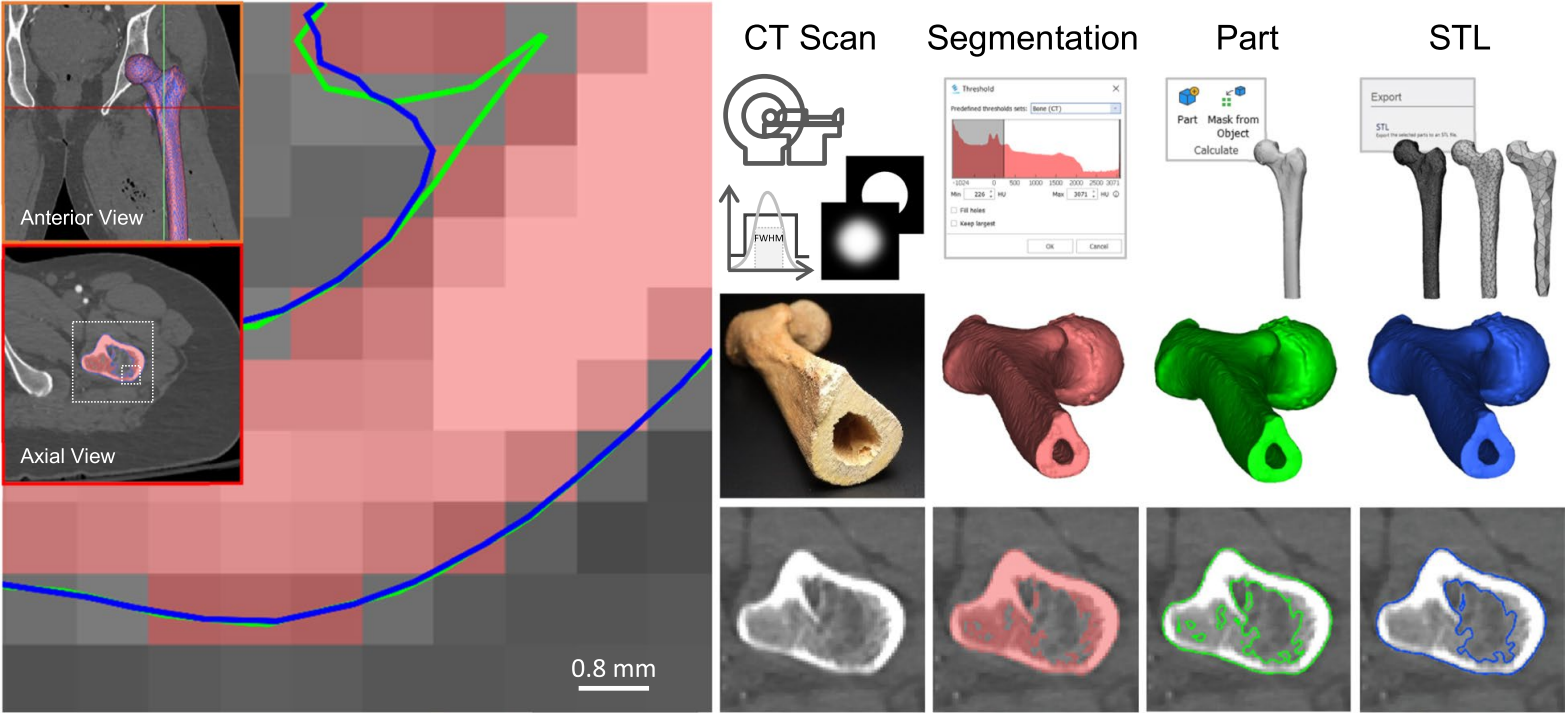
Comparison of 3D model morphology of a femur at high magnification. CT scan data (greyscale) was segmented (red) in Mimics (Materialise), converted to a ‘part (green) and exported to an STL file (blue) after ‘wrapping’ and floating body removal
